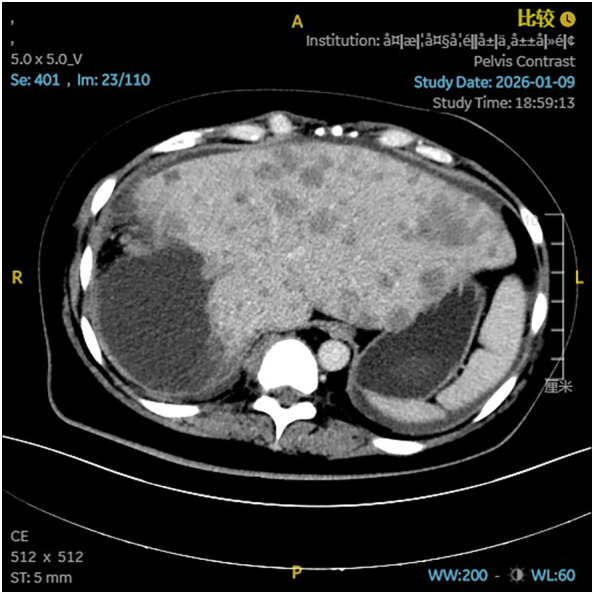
Postoperative computed tomography showing early recurrence in the remnant liver. Contrast-enhanced abdominal computed tomography obtained approximately 1 month after surgery shows multiple recurrent metastatic lesions throughout the remnant liver, consistent with rapid postoperative progression.
